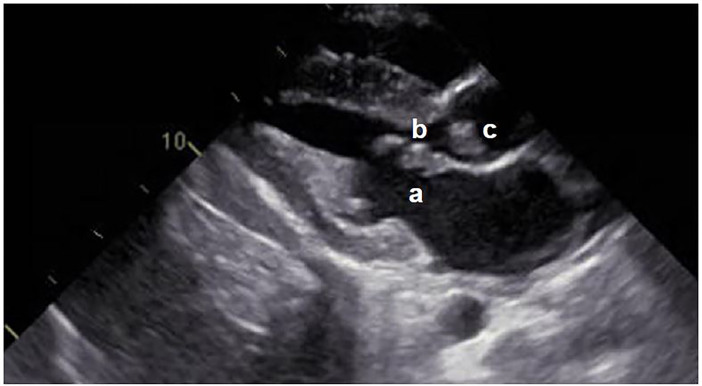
Transthoracic echocardiogram showing focal thickening of the anterior leaflet of the mitral valve (a) with a large perforation (b), as well as increased thickness of the aortic valve with vegetations (c).
